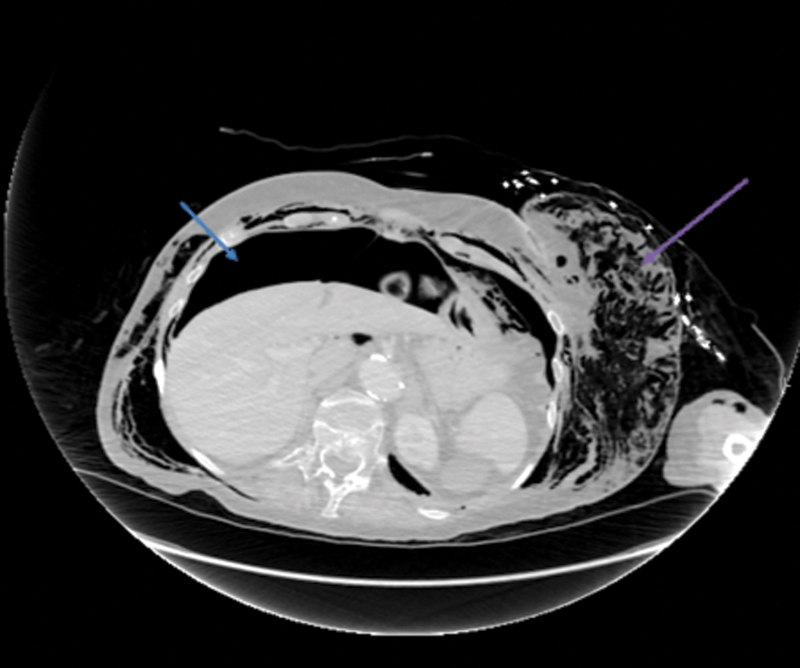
Axial computed tomography image demonstrating free gas in the peritoneal cavity (blue arrow) and subcutaneous emphysema in the left chest/abdominal wall (purple arrow).
